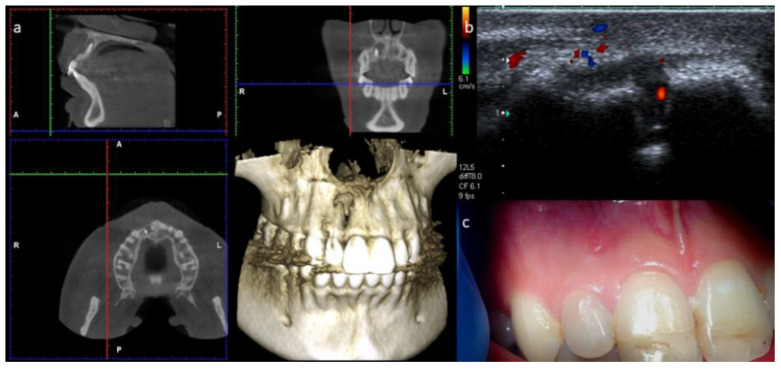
Three-month follow-up. (a) CBCT depicting the extent of the lesion and the extensive bone loss involving the periapical area of teeth 1.2 and 1.1. (b) Color Doppler examination showing the persistence of both the sinus tract and the inflammatory vascular signal within the lesion. (c) Clinical picture showing the persistence of the sinus tract.
